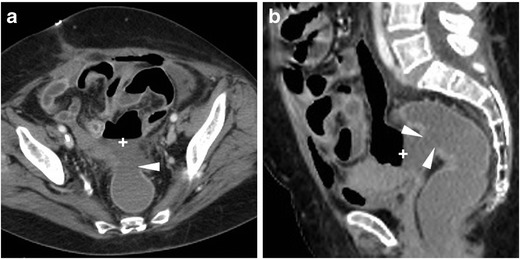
Large-bowel perforation following AH. Post-surgical CT showed a distended rectum and sigmoid, and a clear-cut mural discontinuity (arrowheads) of the anterior aspect of the distal sigmoid colon, from which fluid and air flowed in the peritoneal cavity. Surgical reoperation was necessary
